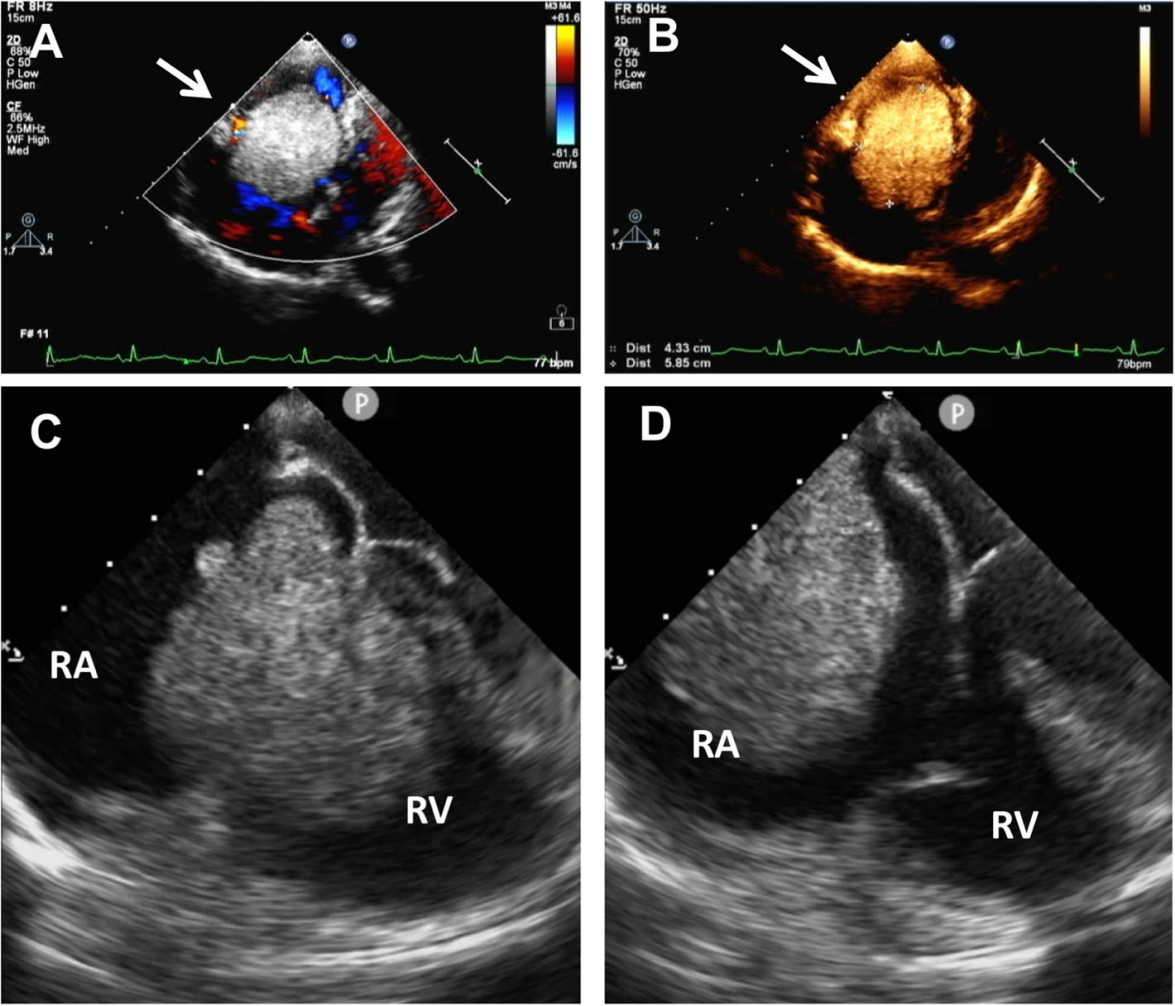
Transthoracic echocardiography showed a giant mass (white arrow) in the right atrium (a-b). Transesophageal echocardiogram clearly showed the mass obstructed into the tricuspid valves and retracted to the right atrium afterwards (c-d). RA: right atrium; RV: right ventricle
